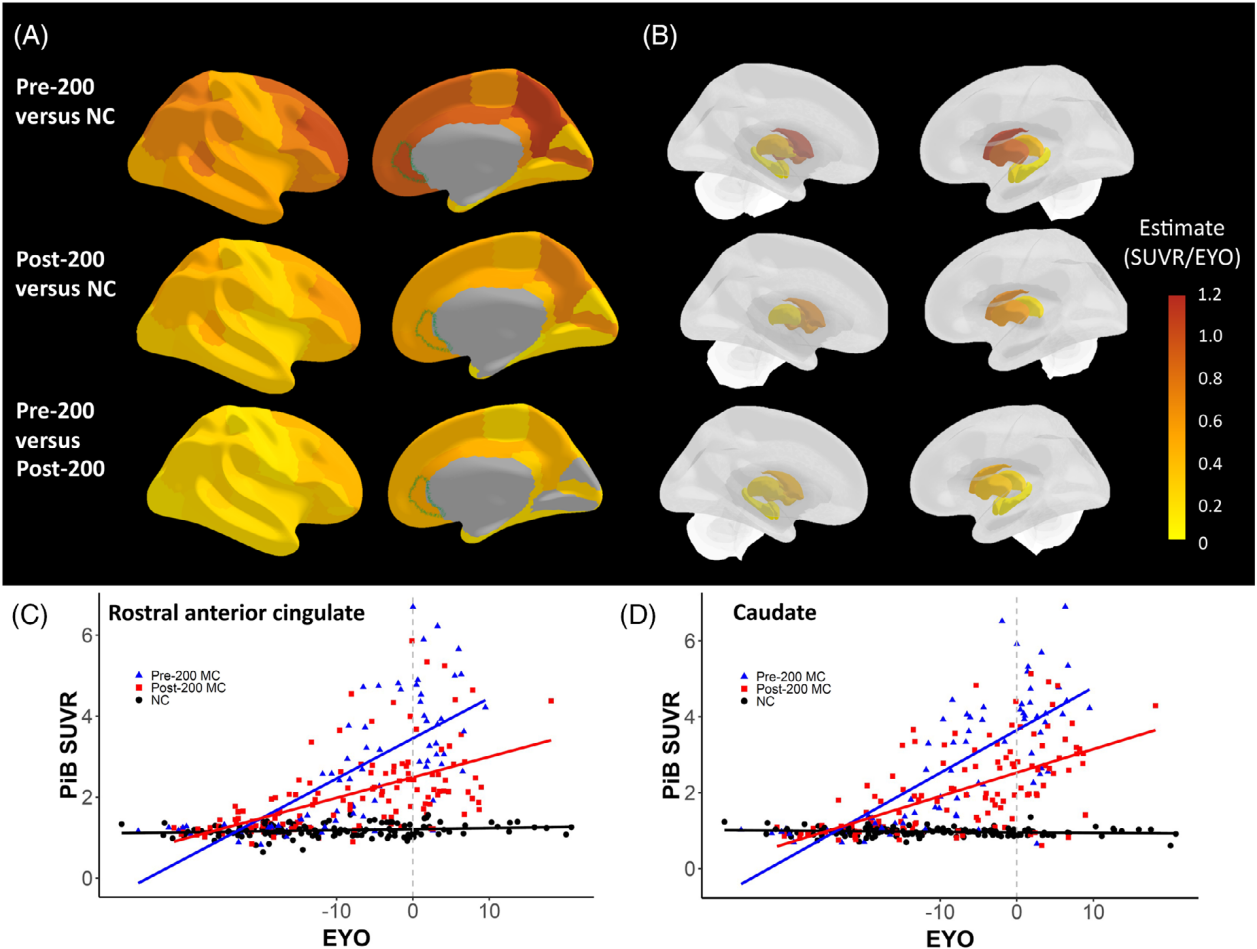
Cross‐sectional regional PiB uptake as a function of EYO. (A and B) Brain maps displaying significant different slope estimates between PiB SUVR and EYO for 34 cortical (A) and subcortical (B) FreeSurfer regions in pre‐200 carriers versus non‐carriers (top row), post‐200 carriers versus non‐carriers (middle row), and pre‐200 versus post‐200 (bottom row). The rostral anterior cingulate (green outline) and caudate showed the strongest divergence between pre‐ and post‐200. (C and D) Plots of cross‐sectional PiB uptake as a function of EYO for pre‐200 carriers (blue triangle), post‐200 carriers (red square), and non‐carriers (black circle) in rostral anterior cingulate (C) and in caudate (D). Color bar = estimates from LME model of PiB SUVR per EYO. EYO, estimated years to symptom onset; LME, linear mixed effect; NC, non‐carriers; MC, mutation carrier; PiB, Pittsburgh compound B; SUVR, standardized uptake value ratio.
